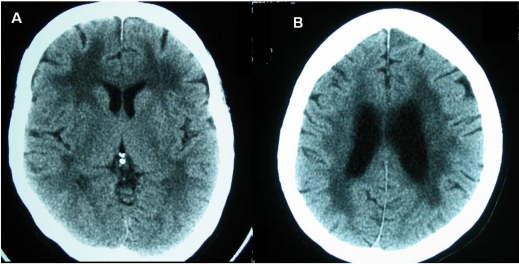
Plain CT brain at the basal ganglia (A) and corona radiata (B) levels showing periventricular white matter lucency in a patient with leuko-araiosis.
